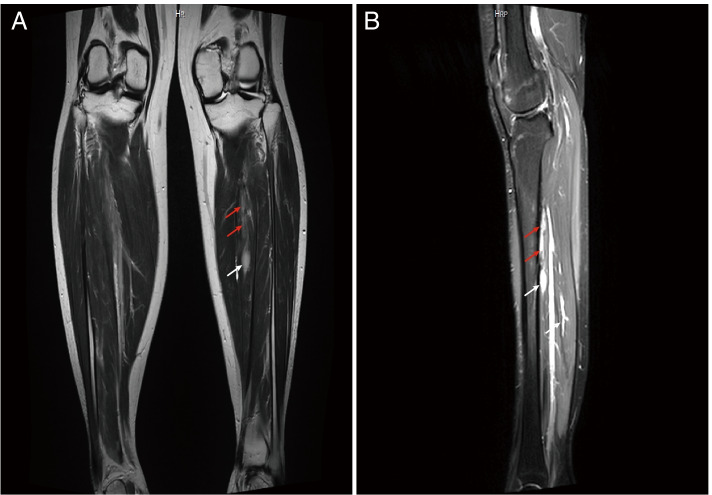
MRI imaging of tumor-induced osteomalacia. MRI shows an elliptic slightly high signal near the cortical bone of the posterior edge of the left middle tibia (1.6*0.7 cm, indicated by the white arrow), and a small nodular, vertical strip abnormal signal outside the bone (1.5 cm, indicated by the red arrows). A Coronal MRI, B sagittal MRI
